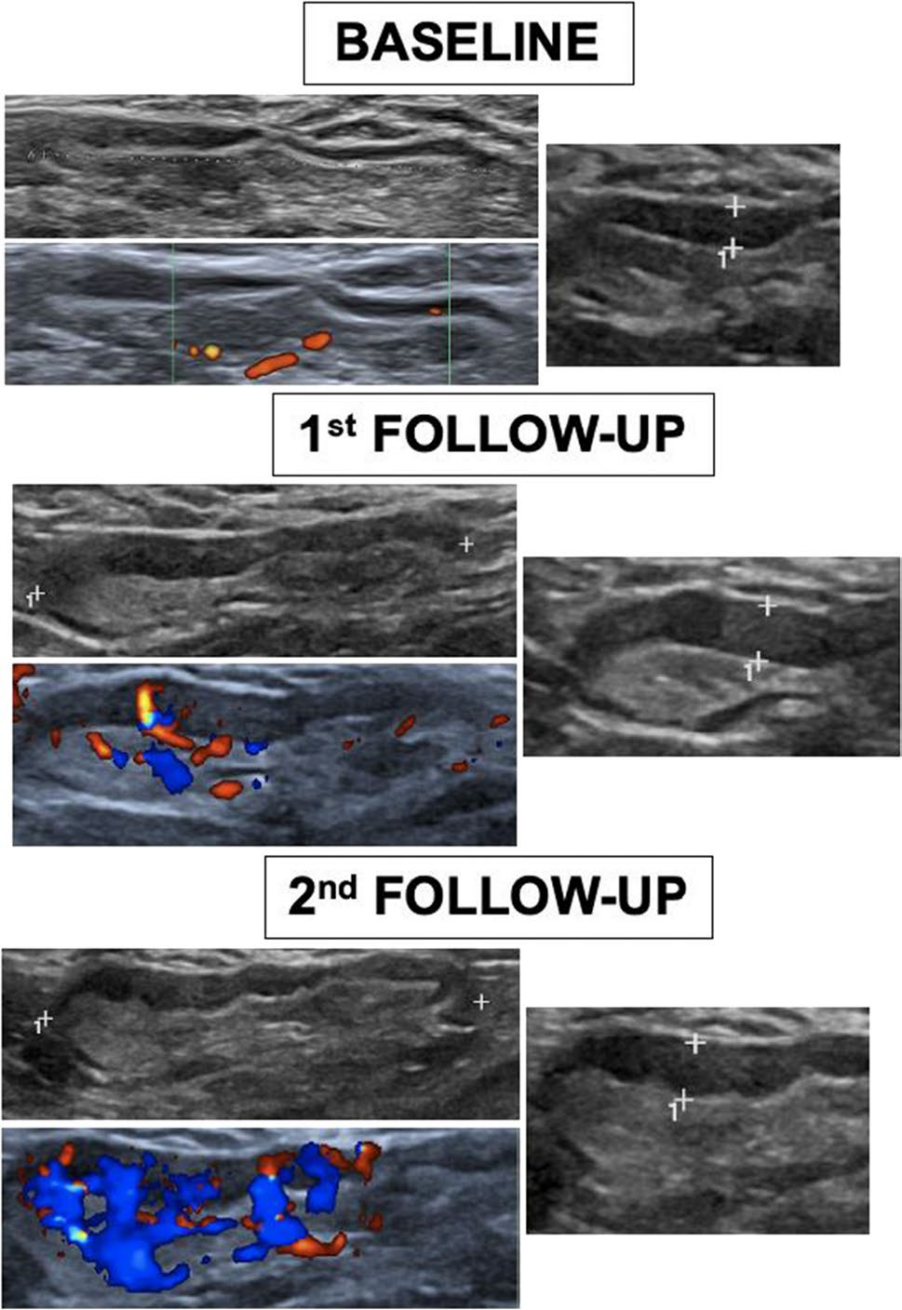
Comparative US images from one volunteer between baseline and the first and second follow-ups showing a significant gradual increment of maximum diameter, cortex, Bedi’s classification, and Doppler scale degree
